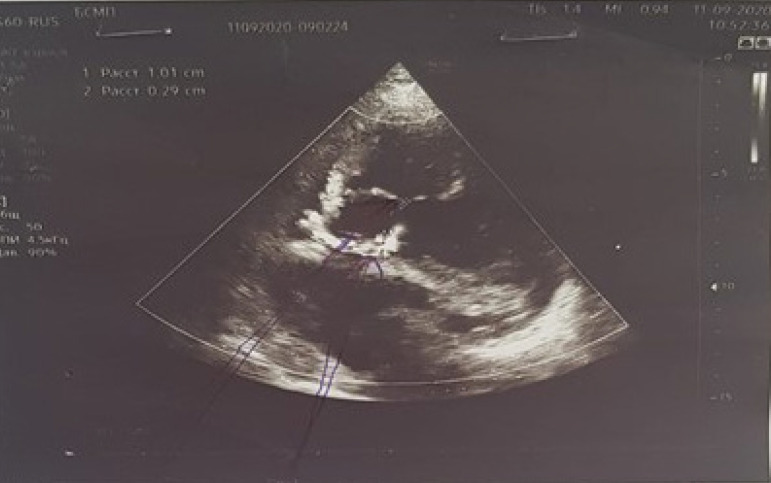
Echocardiography before surgery.
